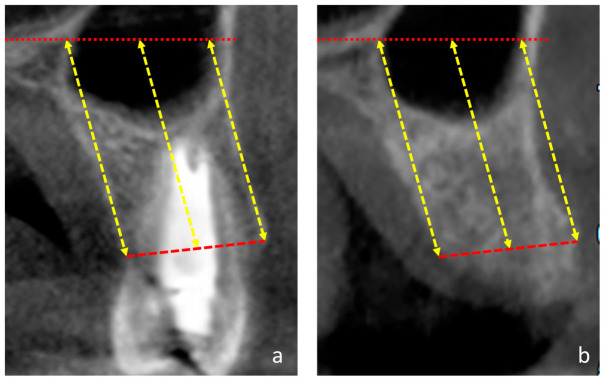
Cone beam computed tomography (CBCT) scans obtained (a) before and (b) approximately 6 months after tooth extraction. One line was drawn parallel to the nasal floor (red dotted line), and another connecting the buccal and lingual crestal bone peaks (red dashed line), representing the crestal bone width. The distance between the two lines was measured, parallel to the bone crest axis, at the buccal, central, and lingual aspects (yellow dashed arrows).
